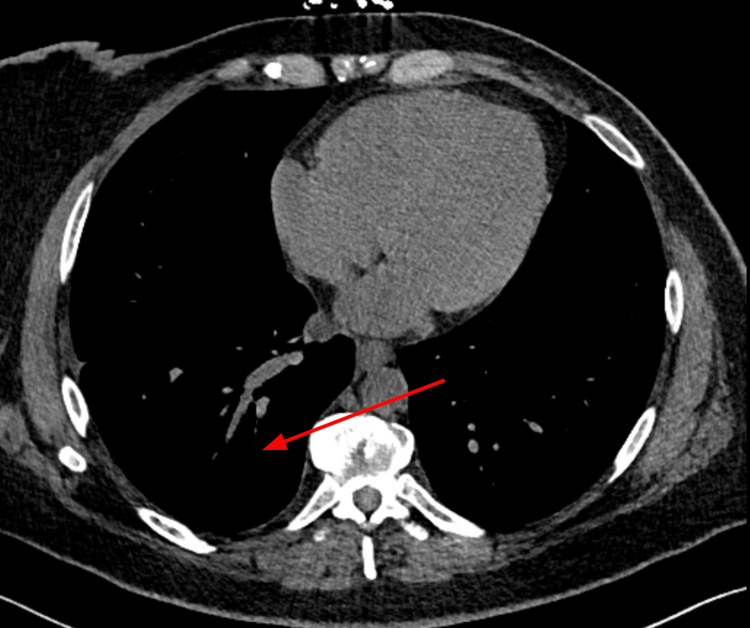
CT chest showing resolution of right pleural effusion. Resolution of right pleural effusion in comparison to Figure 3.
